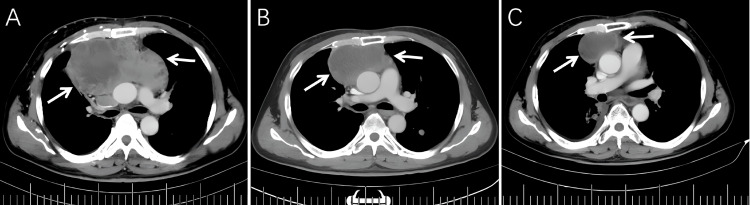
Serial chest CT images (mediastinal windows) demonstrating temporal changes in the anterior mediastinal mass. (A) The thorax CT scan obtained on the first admission showed a mass in the anterior mediastinum with the size of 149 × 73 mm. (B) After chemoradiotherapy, the tumor has decreased in size (94 × 57 mm). (C) Subsequent CT examination after four cycles of combined immunotherapy (sintilimab) with chemotherapy demonstrates further substantial tumor regression, measuring 62 × 42 mm. (White arrows indicate the anterior mediastinal mass.)
